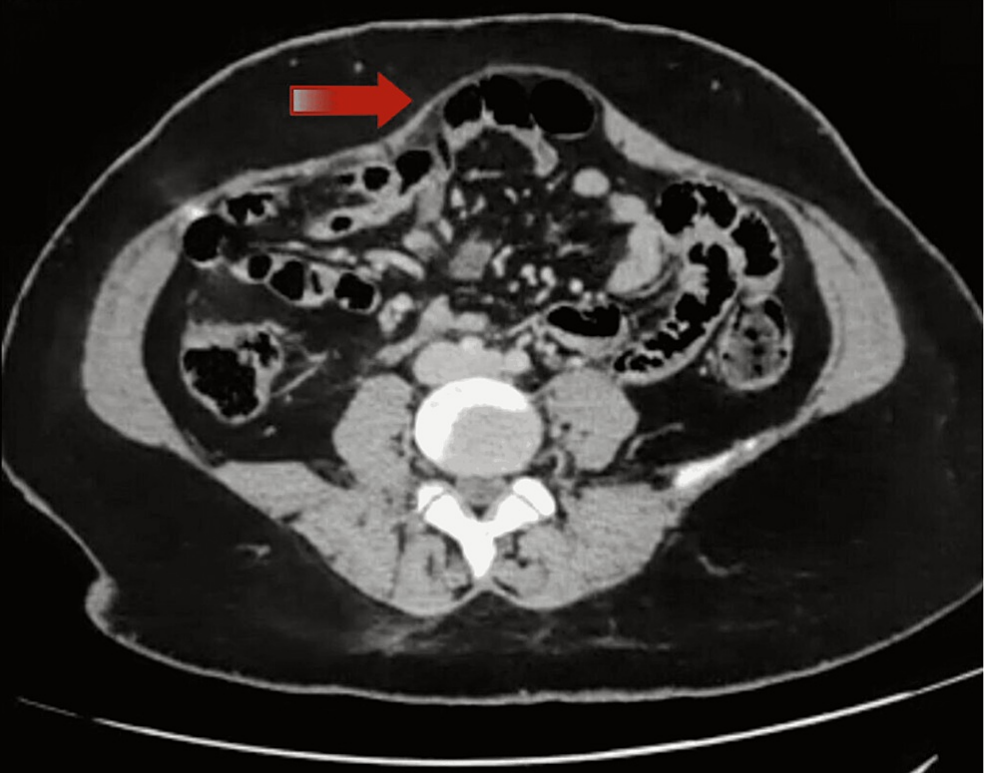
CT scan depicting divarication of recti
